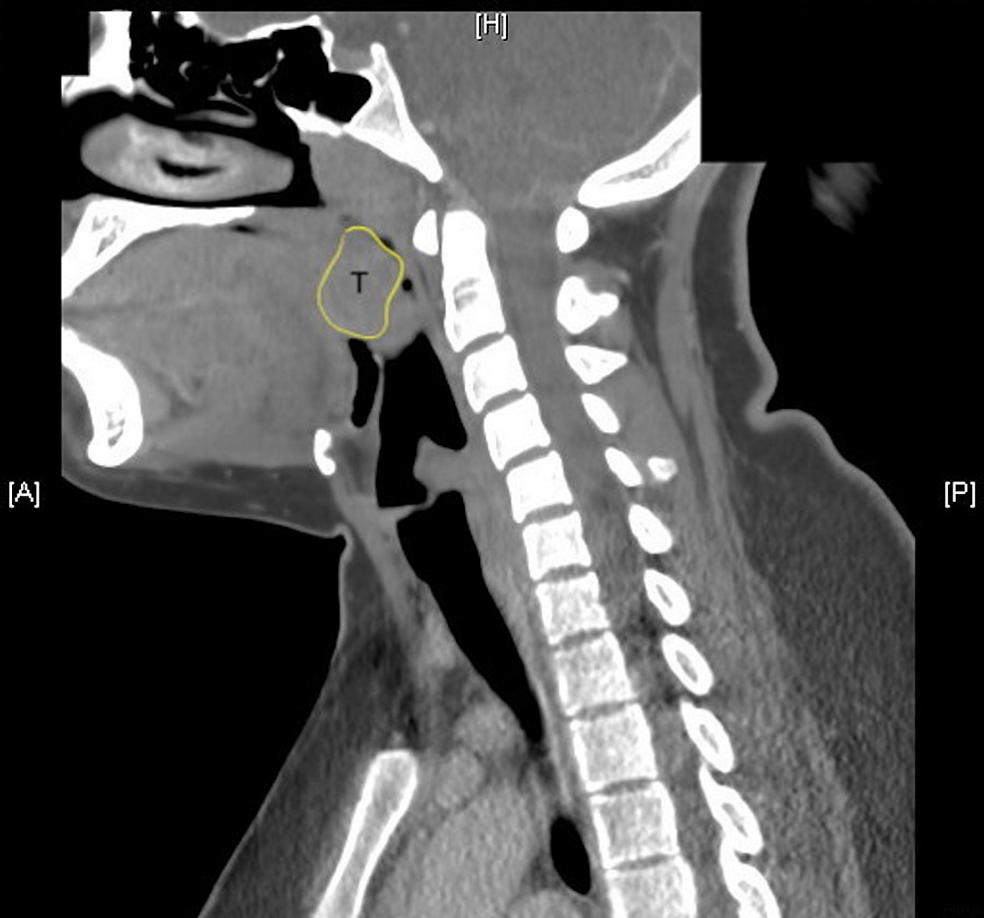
Saggital-view computed tomography image demonstrating tonsillar abscess (T)
